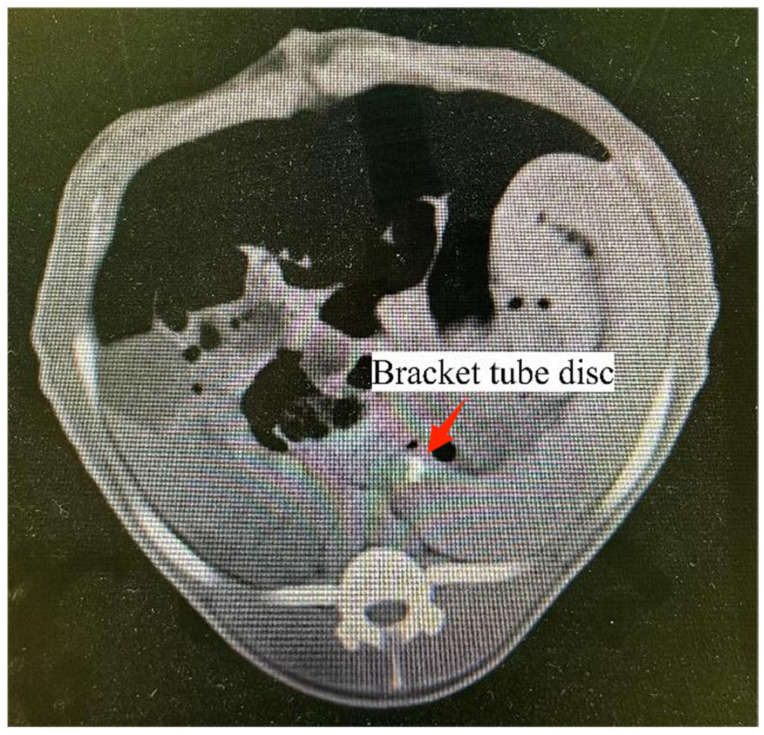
The novel stent was visualized in the second-stage postoperative follow-up CT scan. The stent was in a good position and was visualized because barium sulfate was added to the head of the stent tube.
